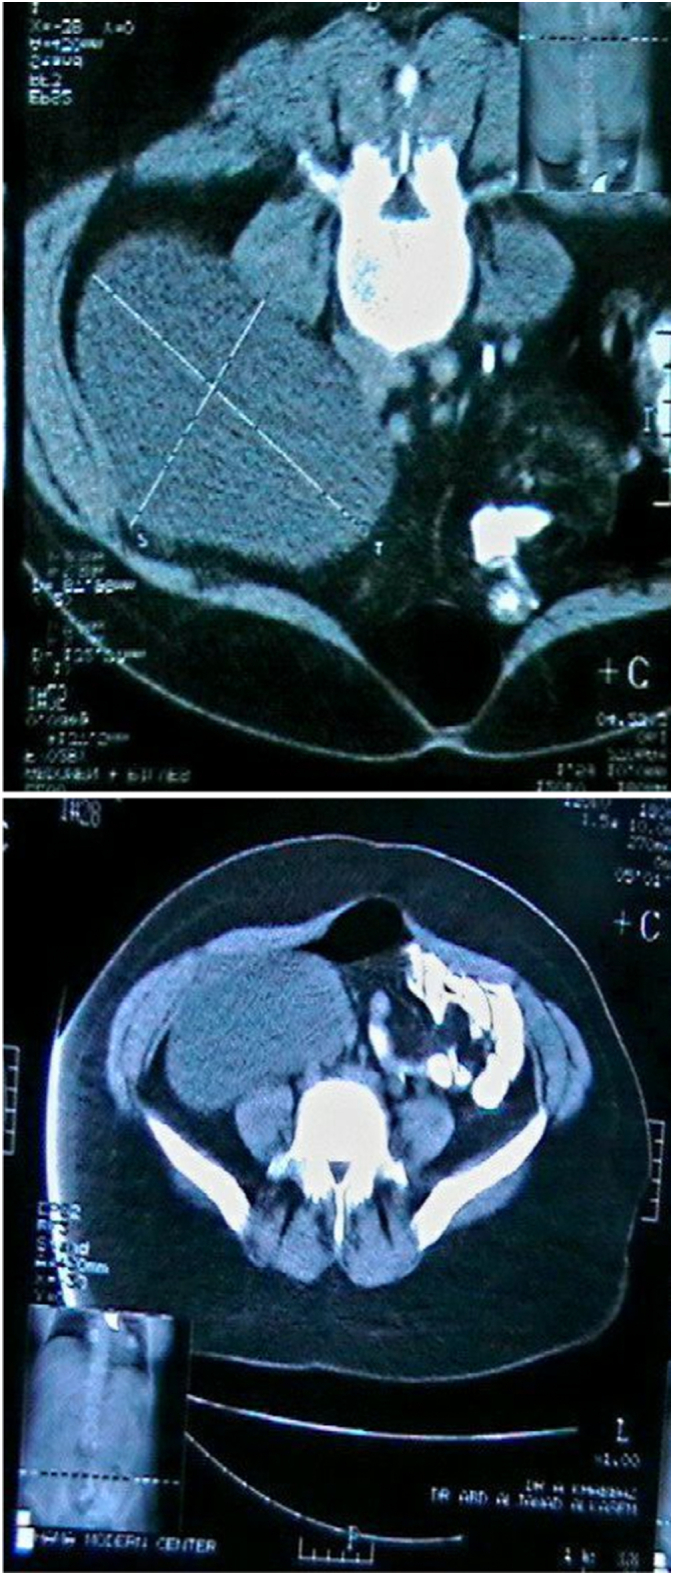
A computed tomography (CT) scan of the abdomen showed a right adnexal mass that was highly probable a serous ovarian tumor.
